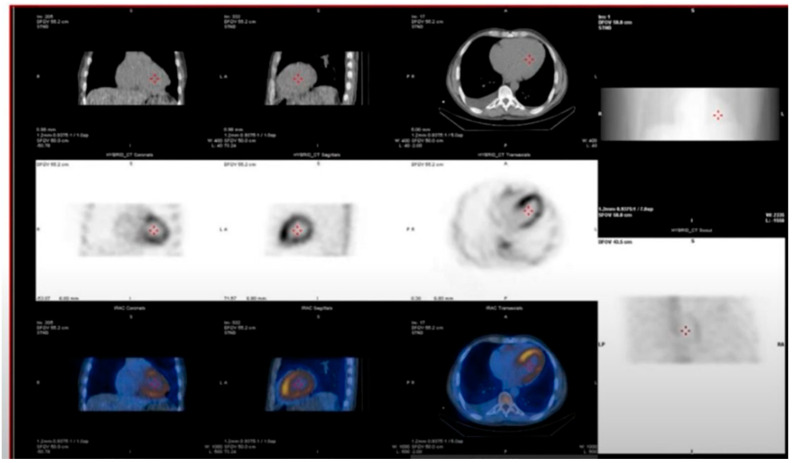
SPECT-CT imaging in cardiac amyloidosis. The middle row and bottom row images demonstrate intense myocardial tracer uptake on SPECT corresponding to the left ventricular myocardium on CT (upper row), with activity equal to or greater than bone uptake. This finding is consistent with a positive ^99m^Tc-pyrophosphate (PYP) scan, characteristic of transthyretin (ATTR) cardiac amyloidosis.
